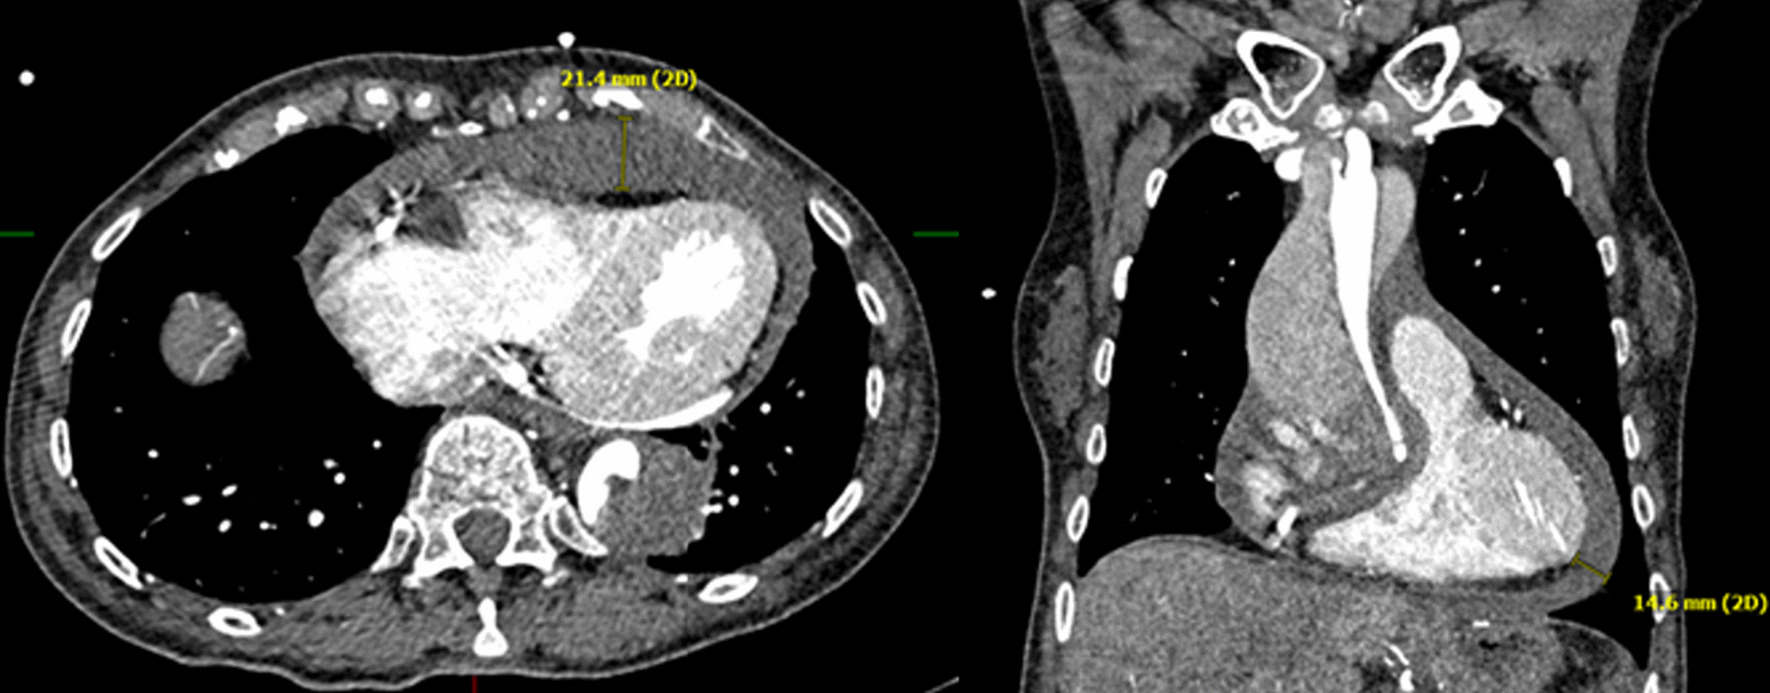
Transversal (left) and coronal (right) CT scan with imaging of pericardial hematoma due to AAAD
